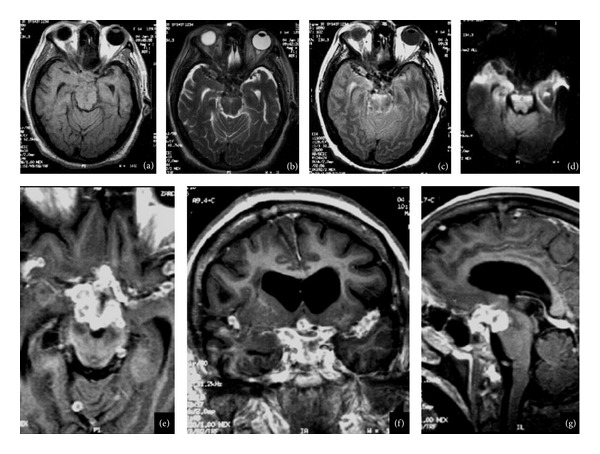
(a) Axial T1-weighted images, (b) axial T2-weighted images, and (c) axial T2- and T2-FLAIR-weighted images after contrast media administration. (e) Axial plane, (f) coronal, (g) sagittal MRI images of 57-year-old woman, six years before she had tuberculous meningitis, obstructive hydrocephalus secondary to multiple coalescent nodular well-defined images; the biggest was localized in the sella, the lesion in contact with the chiasm, and extends to cavernous sinuses. Multiple lesions were localized in basal cisterns and both lateral fissures and the retrosellar extension of the lesions cause brain stem compression.
